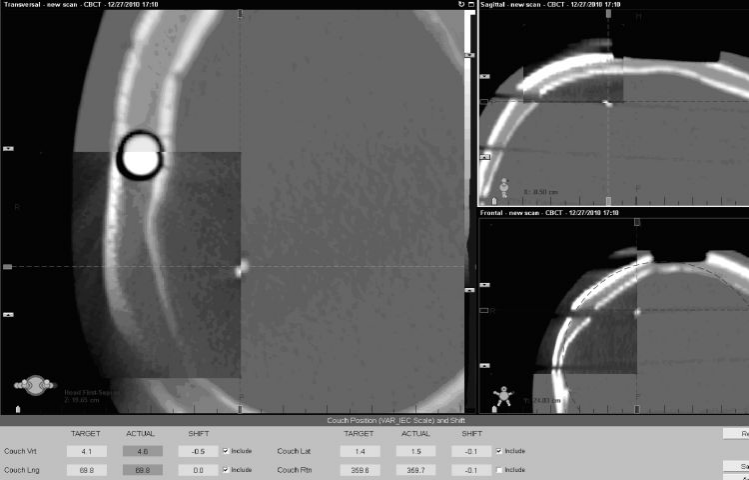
CBCT images at 2.5° tilt. Images inside the spyglass are planning CT
